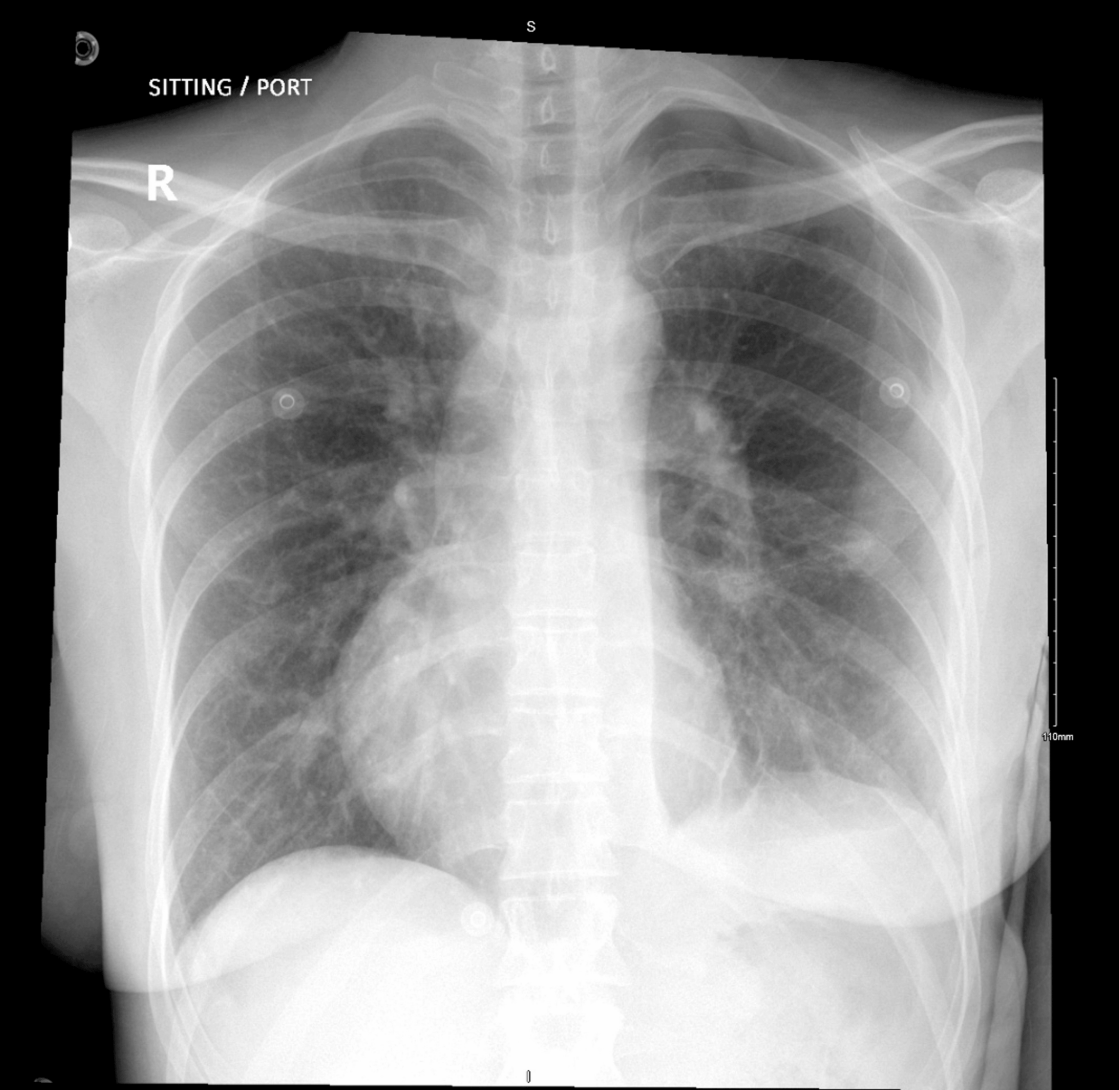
Chest X-ray on postoperative day one, after chest tube removal, showing a well-expanded lung with no complications
